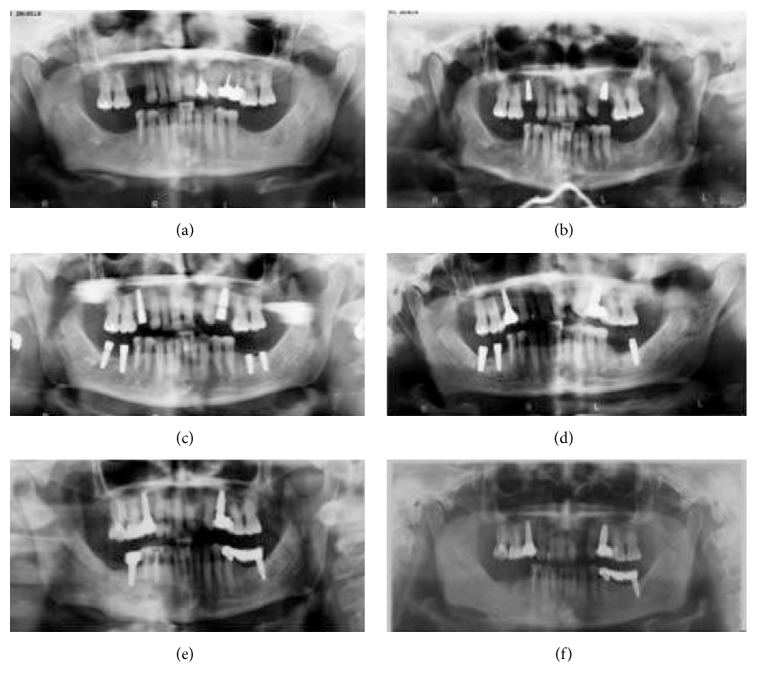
Orthopantomography (OPT) performed on 2009 (a), on May, 2010 (b), on September, 2010 (c), on May, 2011 (d), on August, 2011 (e), and on August, 2014 (f). The last one revealed osteosclerosis, osteolysis, and subperiosteal bone deposition and persistence of extraction socket.
